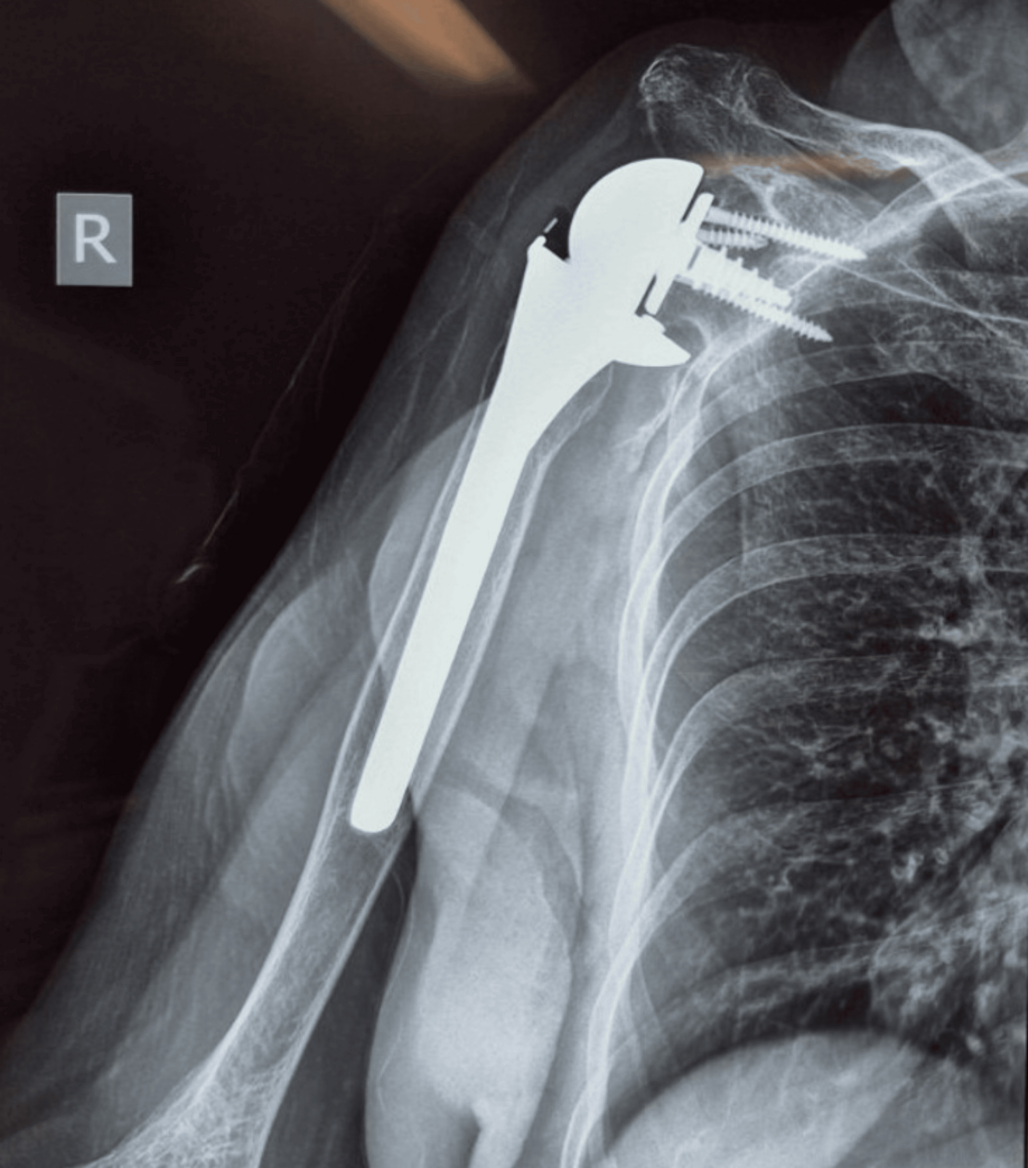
Anteroposterior radiograph of the right shoulder demonstrating a well-positioned reverse shoulder arthroplasty without signs of loosening, instability, or mechanical failure.
